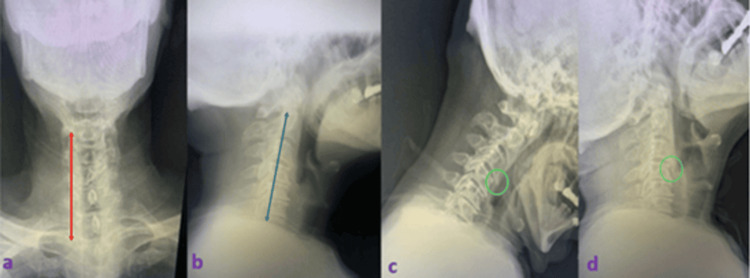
X-ray study showing the primary preoperative radiological assessment to check for dynamic stability in patients who developed cervical disc disease signs and symptoms (a) Antero-posterior, (b) lateral, (c) flexion, and (d) extension images. The orange arrow shows the alignment of vertebrae, the blue arrow indicates the muscle spasm, and the green circle shows minor anterolisthesis.
